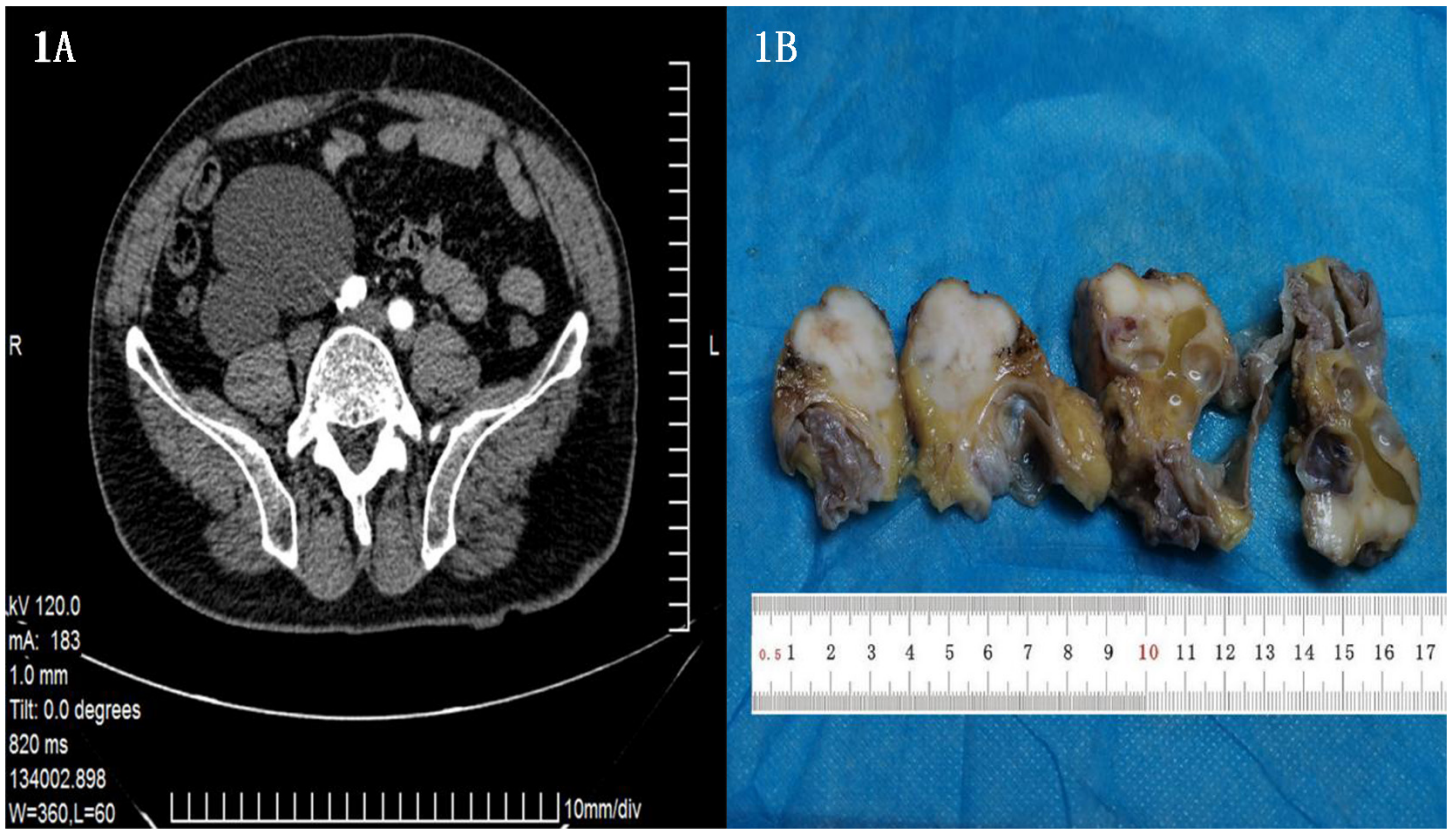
(A) Enhanced CT showing a cystic solid space occupying lesion under the right kidney, with a clear boundary measuring approximately 8.1 × 7.7 × 12.2 cm. (B) A single cystic solid tumor measuring 7*6*4 cm was evident in the retroperitoneum. The cystic area had a diameter of 3 cm, with a smooth inner wall of 0.1 cm thickness. The solid area had diameter of 4 cm, and a cut section was found to be gray white in color, hard, and possessed an intact capsule.
